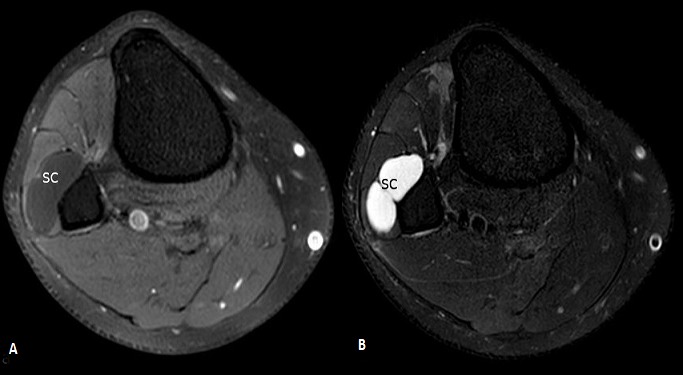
A) axial view of preoperative MRI showing the synovial cyst (SC) hypointense on T1; B) hyperintense on T2-weighted sequence
